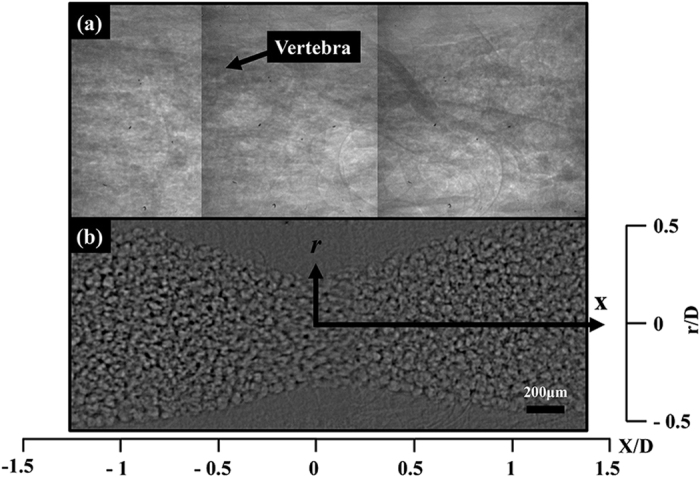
Typical X-ray image of blood flow in the stenosed vessel of S = 36% (a) before and (b) after digital image processing.
